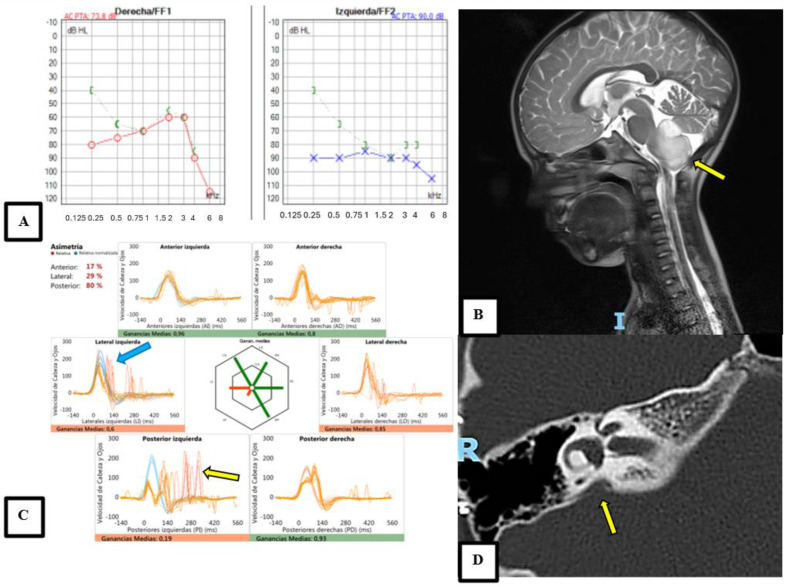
Different complementary tests contributing to the diagnosis of the pathologies included in the sample. (A) Shows a pure tone audiometry of a patient with bilateral Ménière’s disease, exhibiting severe hearing loss with an audiogram pattern typical of the disease, with a bone-air gap at low frequencies. (B) Depicts the only patient with a brain tumor in the bulbomedullary region (yellow arrow) shown on a sagittal brain MRI. (C) Displays a pathological vHIT of a patient with a third window syndrome due to a perilymphatic fistula, showing hypofunctional gains in the left lateral and posterior semicircular canals, with the presence of covert (blue arrow) and overt (yellow arrow) saccades. (D) Presents an axial right temporal bone CT scan of one of the patients with vertigo episodes and severe-to-profound hearing loss due to a dilated vestibular aqueduct (yellow arrow).
